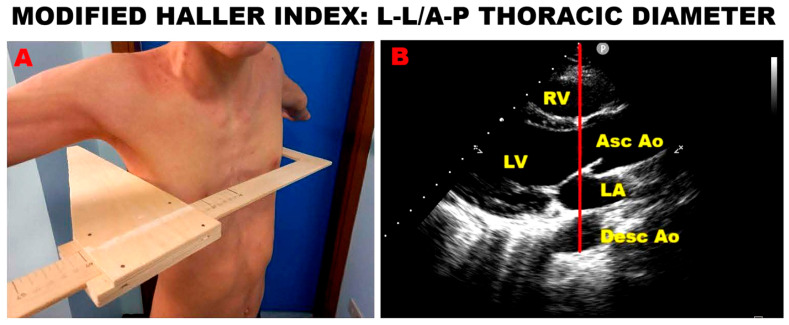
Evaluation of the modified Haller index in a young athlete with pectus excavatum. (A) Measurement of the latero-lateral external thoracic diameter using a rigid ruler and level positioned at the lower third of the sternum. (B) Measurement of the antero-posterior internal thoracic diameter (red line) obtained in the parasternal long-axis echocardiographic view, defined as the distance between the sector apex and the posterior wall of the descending aorta located immediately behind the left atrium. Ao, aorta, Asc, ascending; Desc, descending; LA, left atrium; LV, left ventricle; RV, right ventricle.
